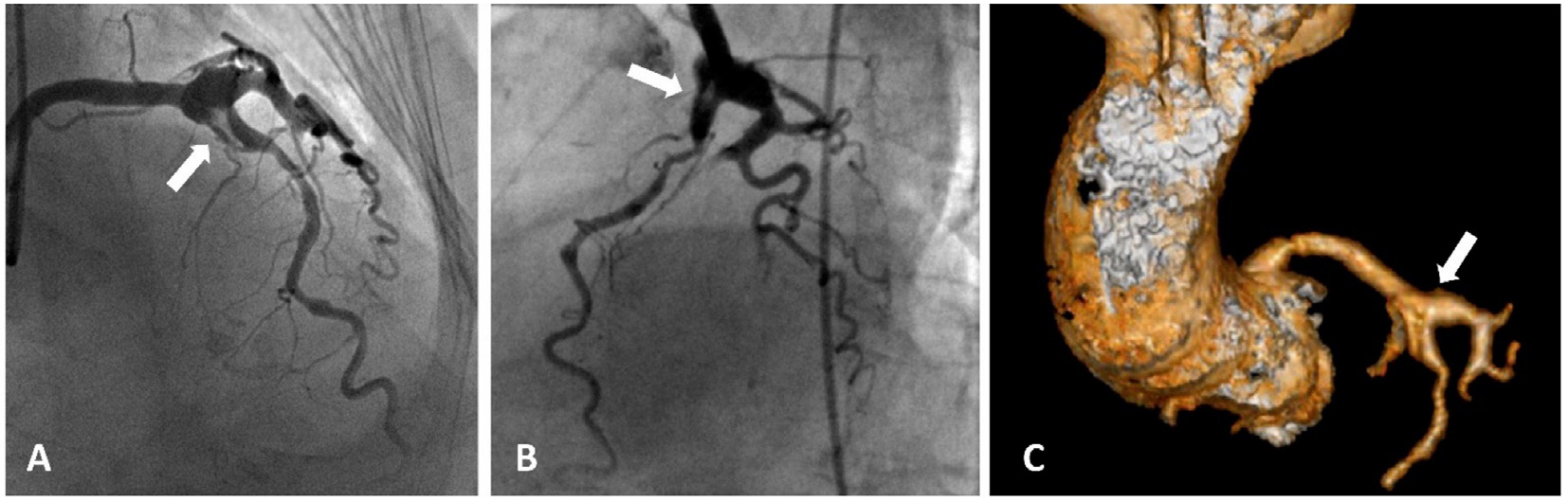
Acute coronary syndrome and coronary artery aneurysms. A patient presented with acute coronary aneurysm. (A,B) Coronary angiography revealed an aneurysmal dilatation of the ostia of the left anterior descending (LAD) artery and left circumflex artery, with a thrombus image (arrow heads) in the LAD artery along with a significant stenosis in the proximal part. Control angiogram failed to show any reduction in the thrombus after 48 h of tirofiban infusion, and the patient was referred to the surgery. (C) Aneurysmal dilatation can also be seen in computed tomography images.
